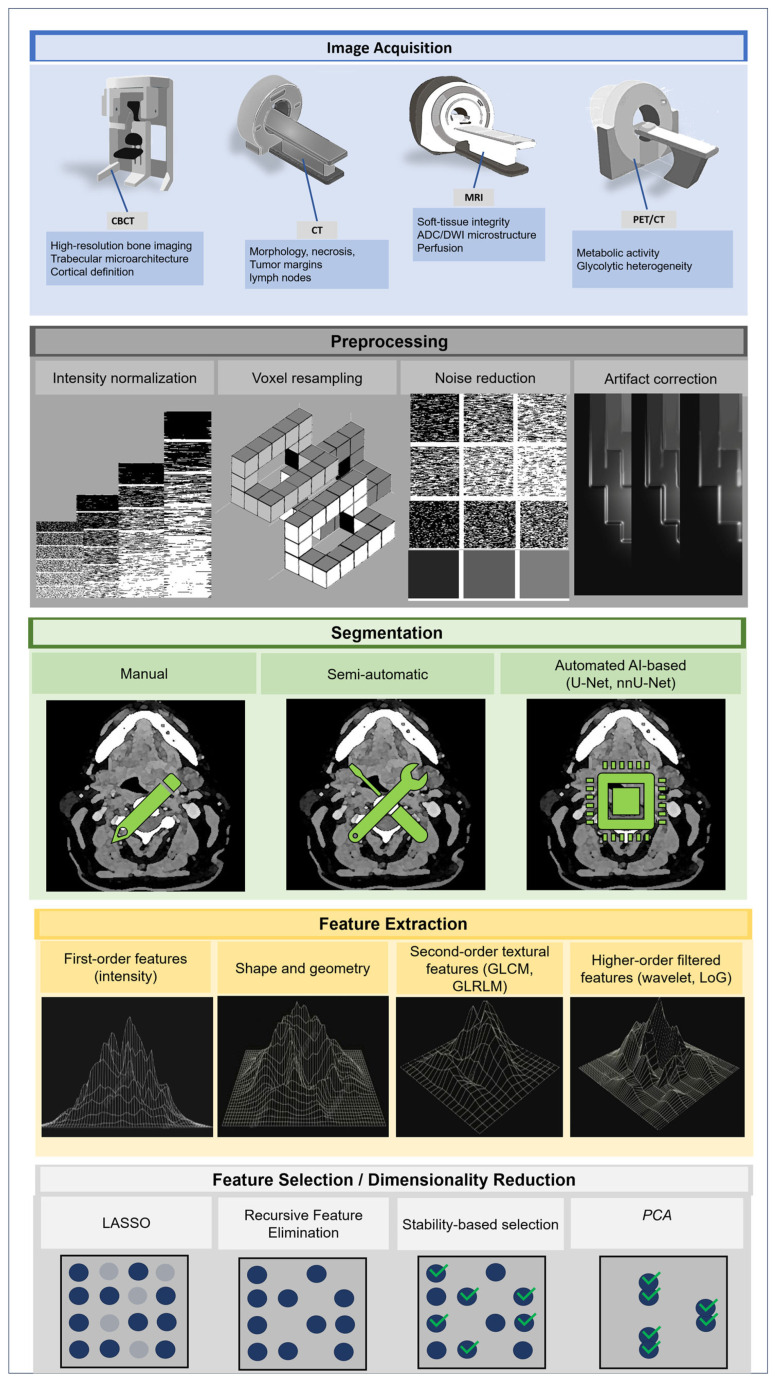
AI-integrated radiogenomic framework for OPSCC. Multimodal imaging features from CT, MRI, PET/CT and CBCT are combined with clinical and genomic information through AI-based models to generate biologically informed predictions.
